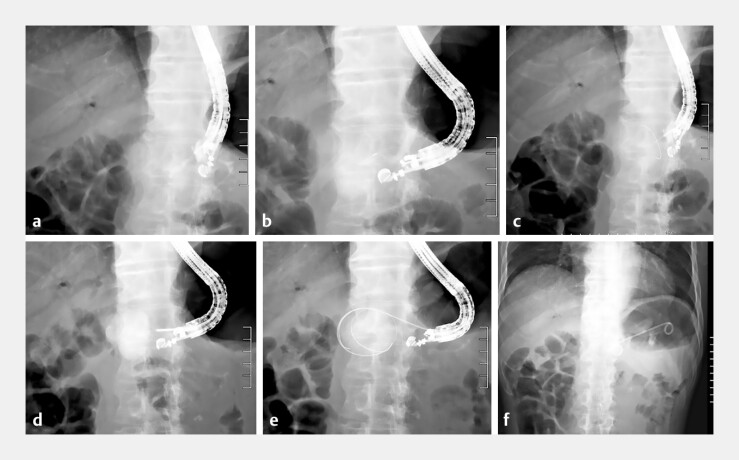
Endoscopic ultrasound-guided rendezvous technique and EUS-guided pancreatic fistula drainage.
